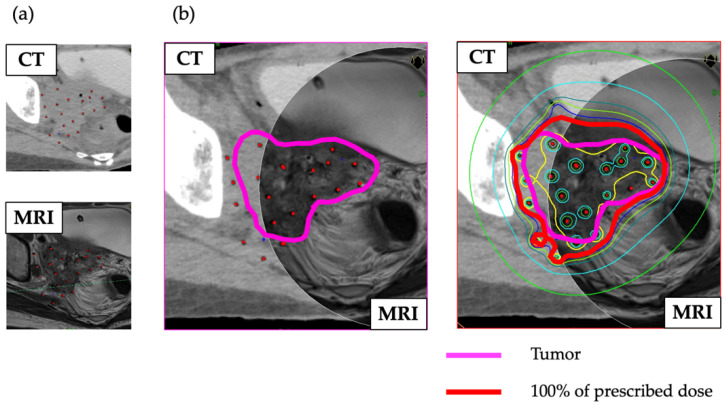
(a) CT and MRI of a patient evaluated after implantation. (b) Combined CT and MRI of the same patient enabled clear visualization of the tumor contours (pink line). The HR-CTV was mostly covered by the 100% prescribed dose (red line).
